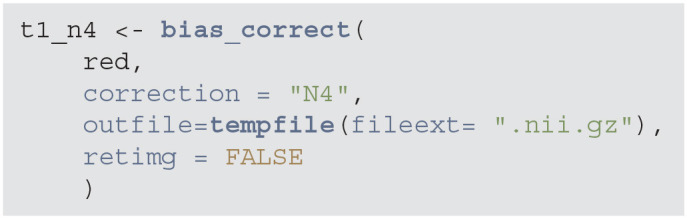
Once images are bias field corrected, we apply brain extraction using a form of multi-atlas label fusion (MALF) (Wang et al., 2012). MALF uses a collection of previously labeled brain images (atlases), aligns the T1-weighted image to each atlas, and obtains a labeled T1-weighted image for each registration. These labels are then combined, or fused, into one labeled map of the processed T1-weighted image. This approach is implemented using the malf function from the malf.templates package, which includes the templates from the 2012 MICCAI Multi-Atlas Labeling Challenge (Landman et al., 2012). Figure 2B displays the T1 image overlaid with the estimated brain mask using this approach indicating that the brain tissue is well estimated and extra-cranial areas are excluded.
